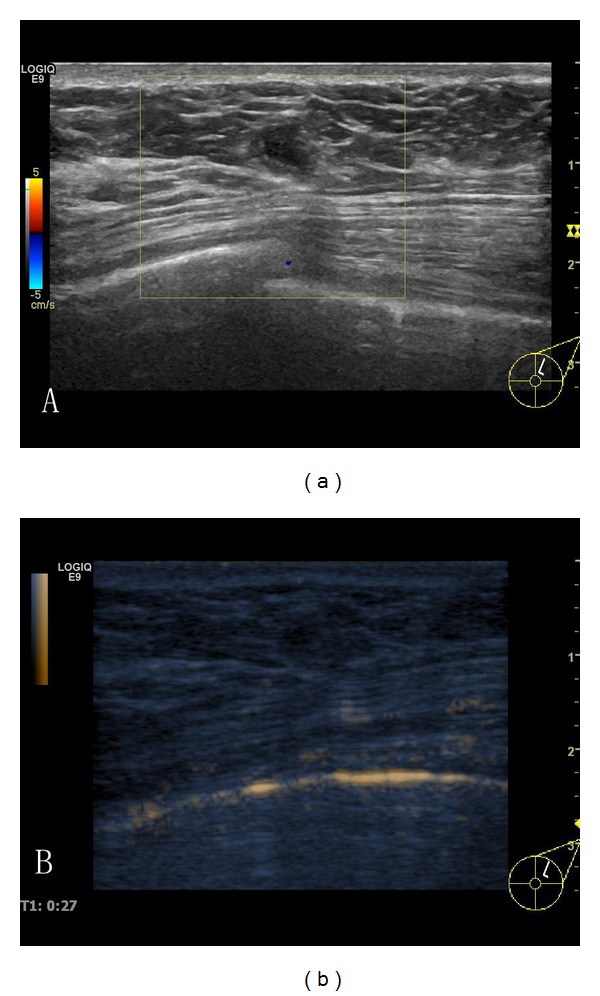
A sonogram of a right breast from a 42-year-old woman is shown. (a) There was a solid hypoechoic nodule of 5 × 4 mm at 12-1 o'clock in the right breast, with a circumscribed margin and defined, irregular shape, with a heterogeneous internal echo and nodular posterior echo enhancement. CDFI (color Doppler flow imaging): there were no color flow signals in and surrounding the nodule. Ultrasound diagnosis: BI-RADS 4a. CEUS (b): there was no significant enhancement in and surrounding the whole of the nodule. CEUS diagnosis: BI-RADS 3. Pathology: hyperplastic disease.
